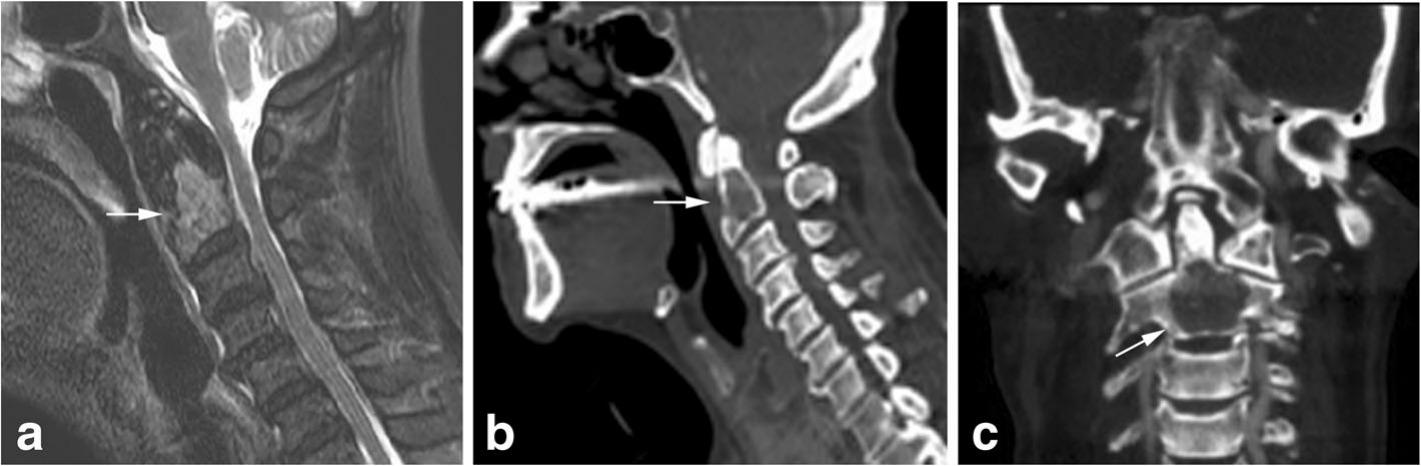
MRI of the cervical spine (a) shows a metastatic lesion in the vertebral body of C2 without spinal cord compression. The CT-scan in lateral and frontal view shows an osteolytic lesion (arrow) in the base of C2 (13,6 mm × 20 mm × 20,9 mm) in the same patient (b and c) (Case 3)
